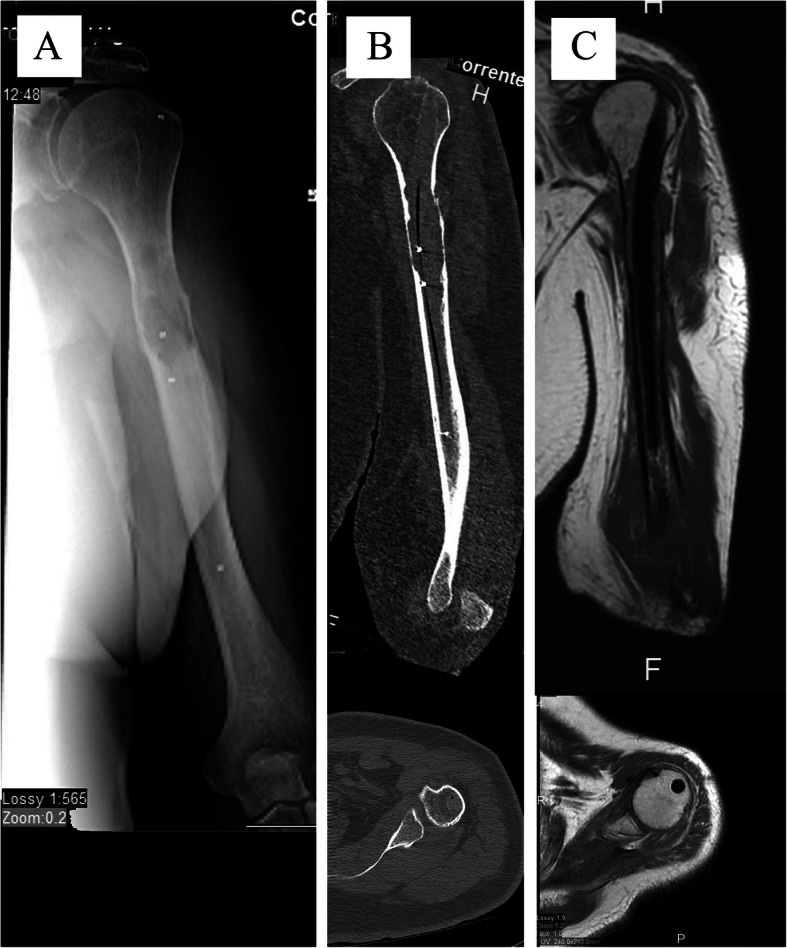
a: X-ray showing the IS-PBSS inside the humerus (the first nails only had a few markers and no information was available regarding the correct expansion; the latest models present a spiral marker which allows to view the correct expansion; b: the same patient’s CT-scan; the complete absence of artifacts enables easy detection of possible local recurrence and a better radiological irradiation; c: the corresponding MRI: note the absence of artifacts in this case also
